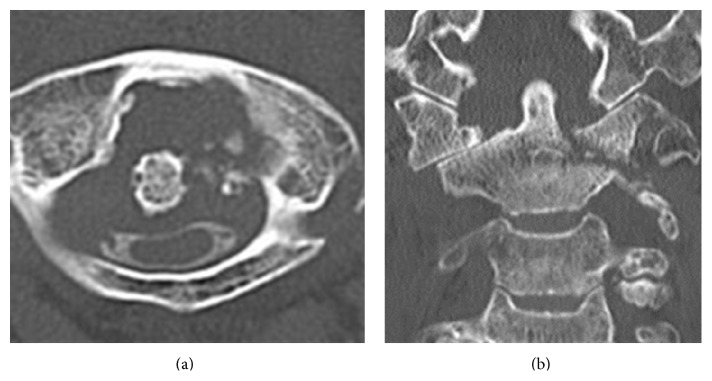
Computed tomography images after myelography showing destruction of the atlantoaxial joint on the left side and compression of cervical spinal cord. (a) Axial view. (b) Coronal view.
